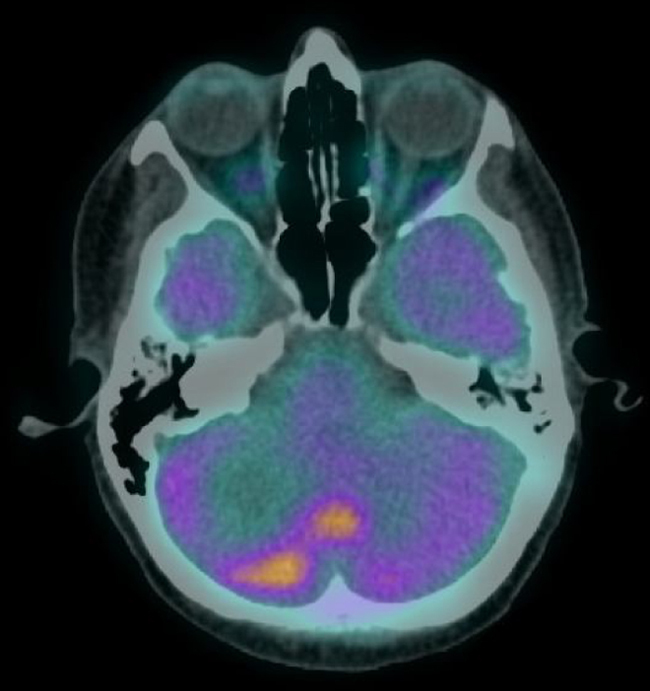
FDG-PET/CT showing FDG hypermetabolism in the right hemisphere and vermis of the cerebellum suggestive of secondary involvement.
